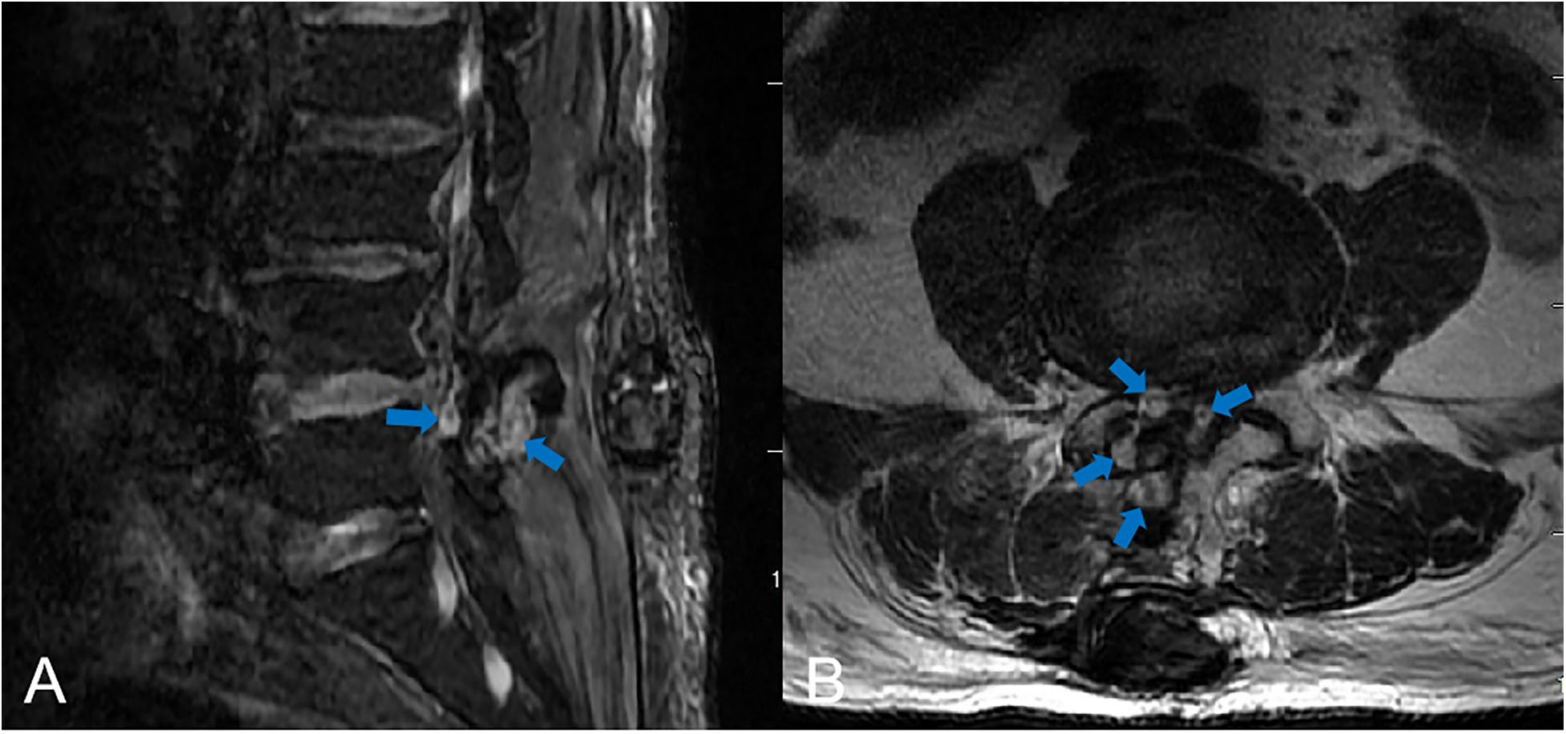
Postoperative MRI revealed a lumbar postoperative epidural hematoma. (A) Sagittal and (B) axial images demonstrated that in this fat-suppressed T2-weighted sequence, the epidural hematoma appears hyperintense (indicated by blue arrows).
